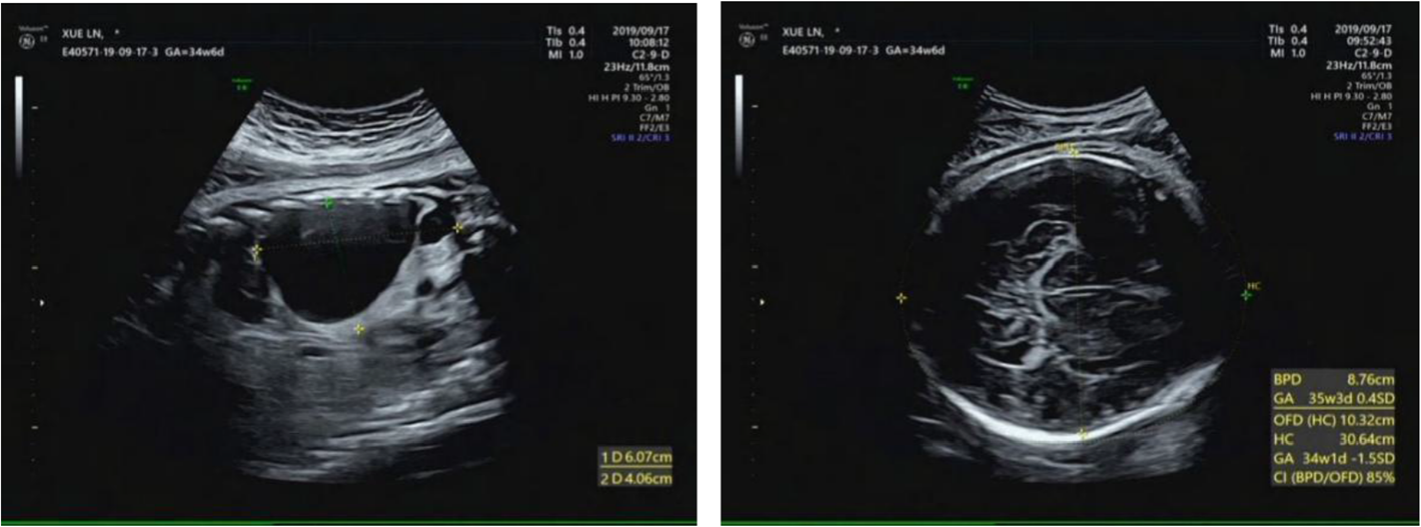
Antenatal ultrasound scan at 33 + 1 weeks gestation, and the ultrasound scan detected an echo-free cyst (6.1 cm × 4.1 cm × 5.9 cm) with a head circumference of 30.6 cm and a CVR of 2.52
